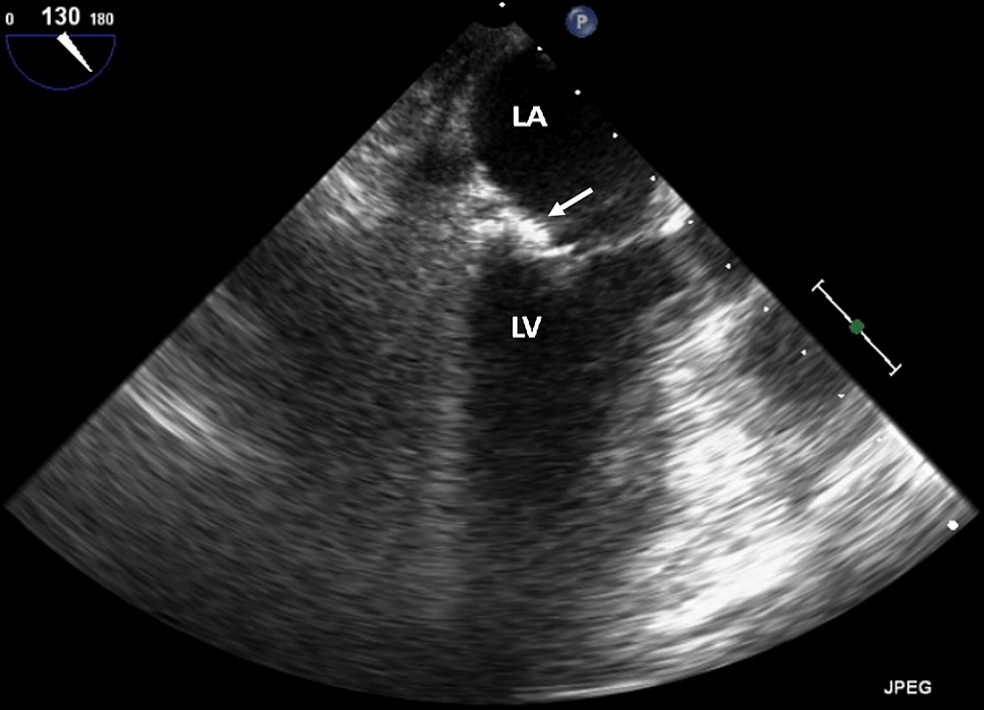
Transesophageal mid-esophageal view at 130 degree orientation with preferential view of the posterior aspect of the mitral valve simply showing showing MAC (white arrow). LA = left atrium, LV = left ventricle, MAC = mitral annular calcification.
